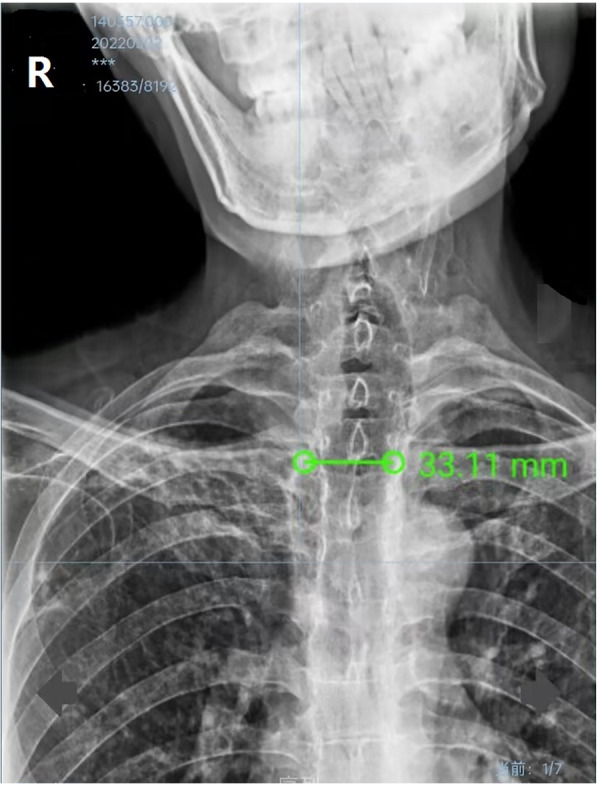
Preoperative thoracic digital radiography image showing that the transverse diameter of the trachea was 33 mm on the level of 2 cm above the aortic arch.
